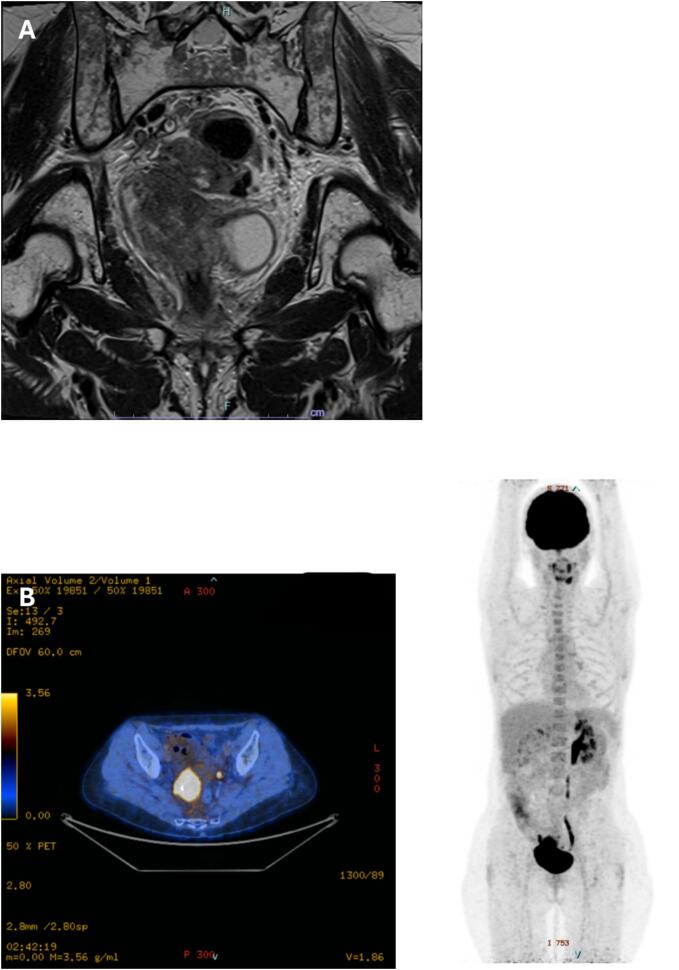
Pre-operative imaging assessment with pelvic MRI (A) and PET-CT (B). A. MRI in axial plane showing the tumor with the lateral involvement. B. PET-CT showing the hypermetabolism of the tumor.
